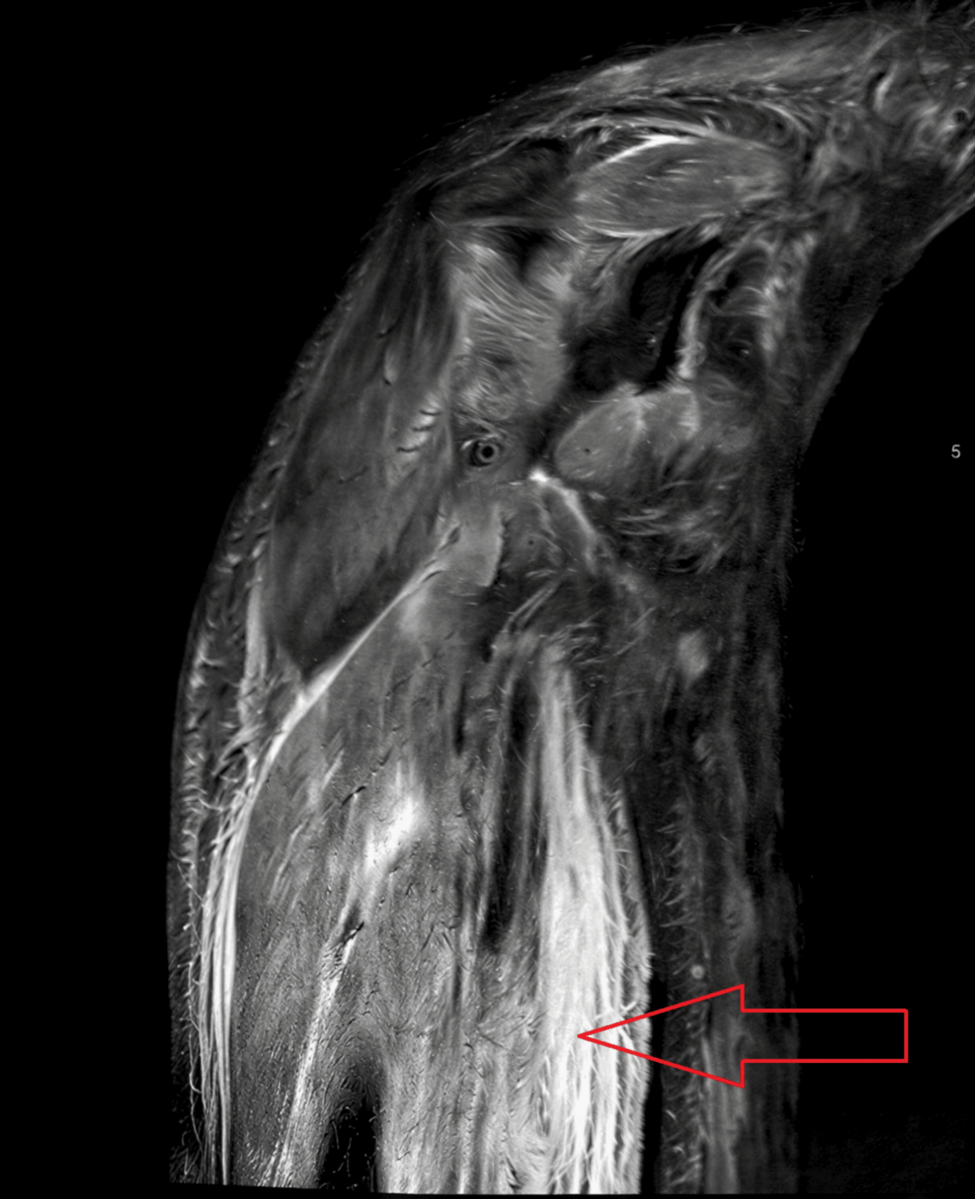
MRI image of the patient's right upper arm, demonstrating changes in keeping with myositis, showing marked involvement of the proximal muscles
